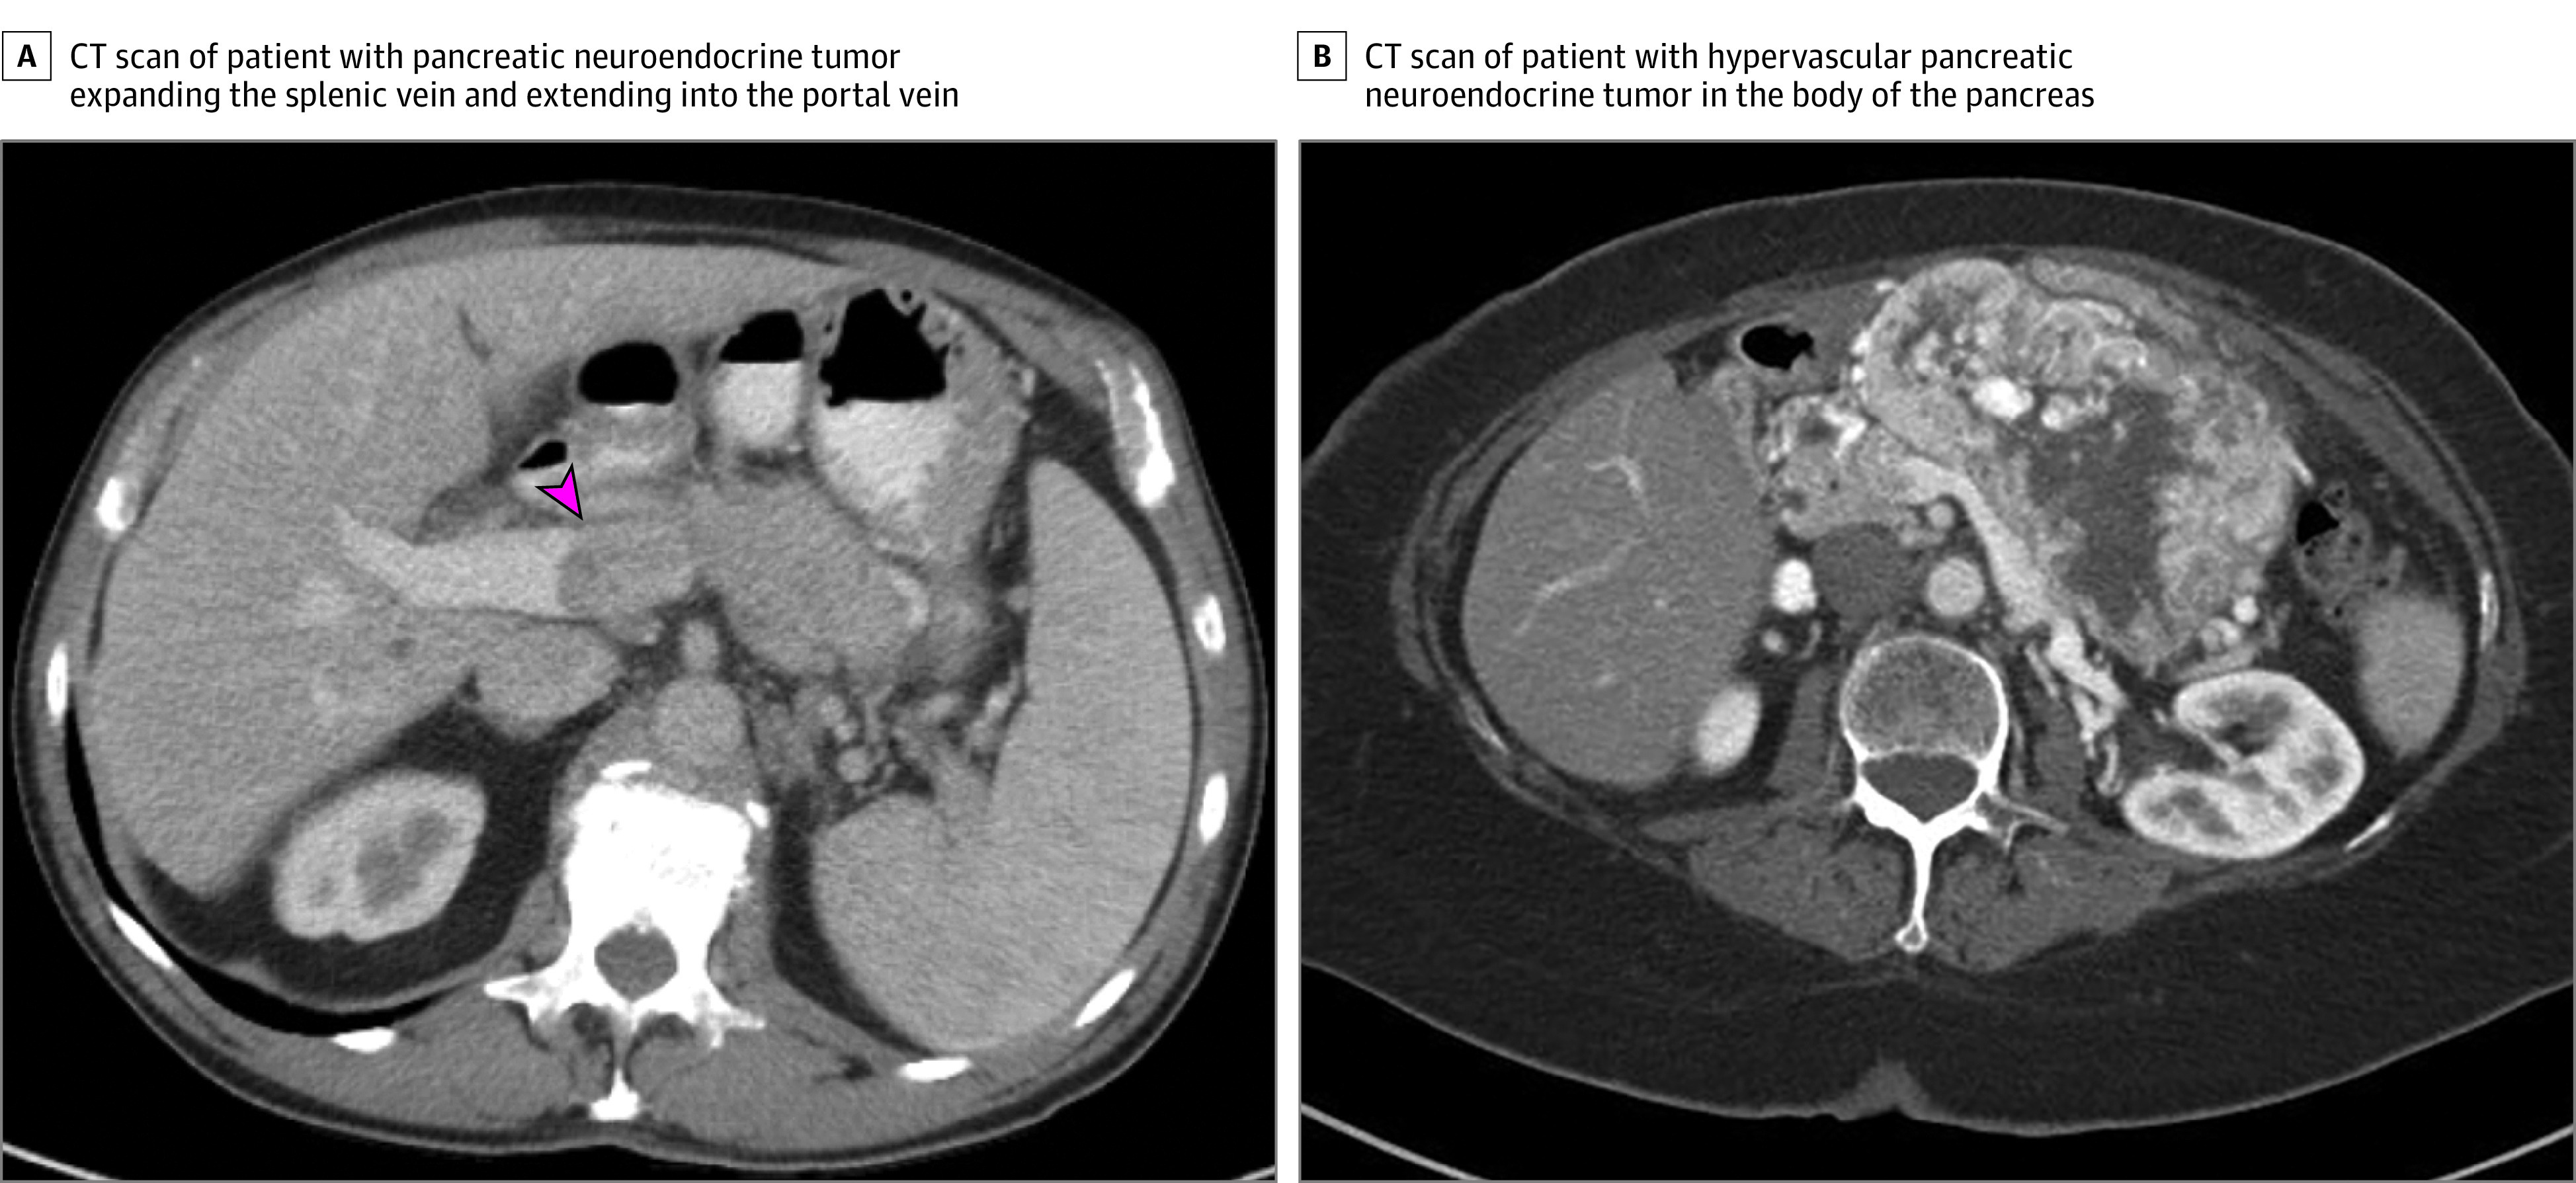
Two Examples of Patients With Locally Advanced Pancreatic Neuroendocrine Tumor Included in This Study A, A computed tomographic (CT) scan showing a patient with an 8 × 2 cm pancreatic neuroendocrine tumor expanding the splenic vein and extending into the portal vein (arrow); B, A CT scan showing another patient with an 8 × 12 cm hypervascular pancreatic neuroendocrine tumor in the body of the pancreas causing mass effect on the splenic vein and porto-splenic confluence.
